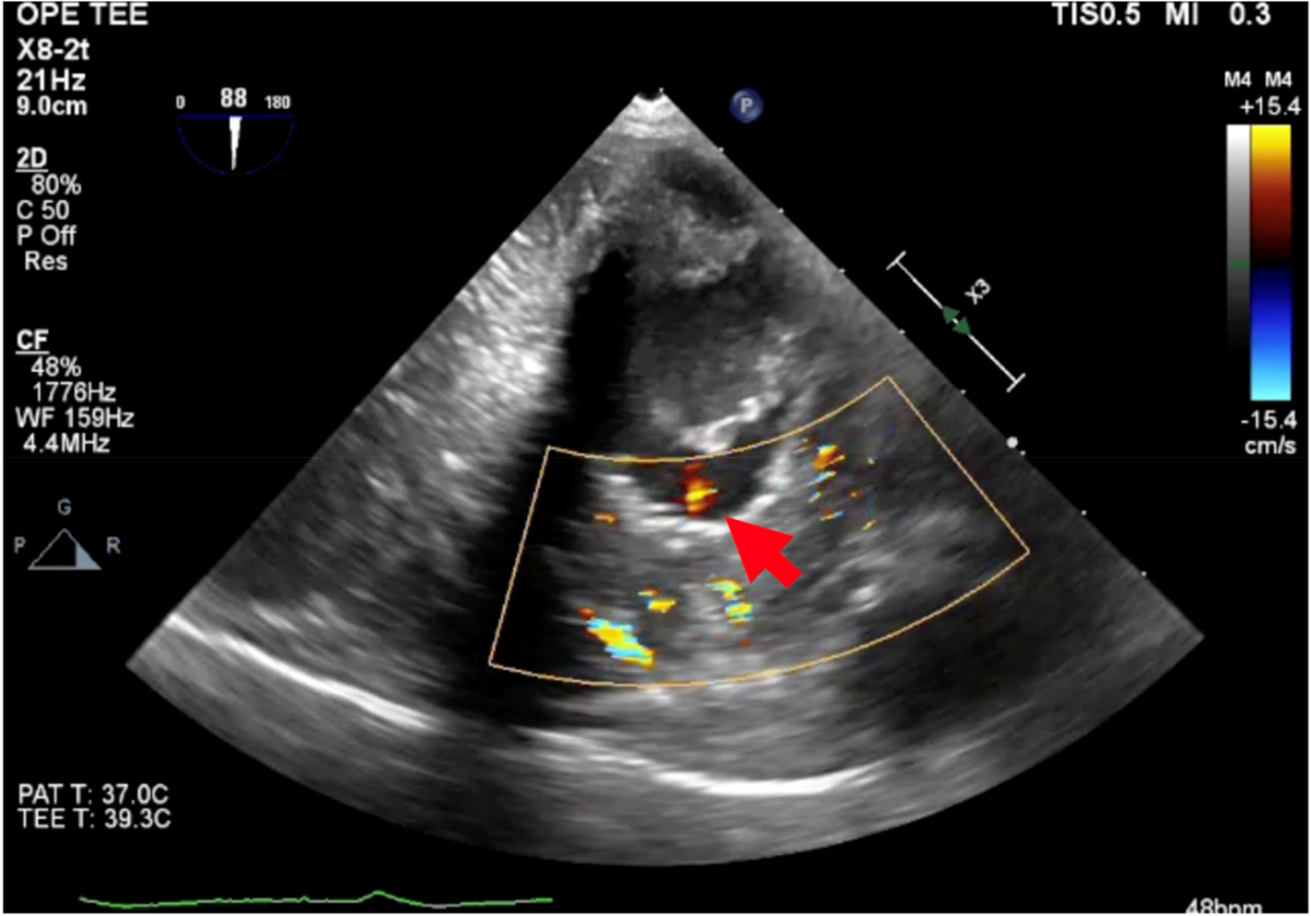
Transesophageal echocardiography during the infusion of terminal warm blood cardioplegia. A coronary artery fistula was detected at the anterior left ventricle wall by transesophageal echocardiography on the trans-gastric short-axis view
